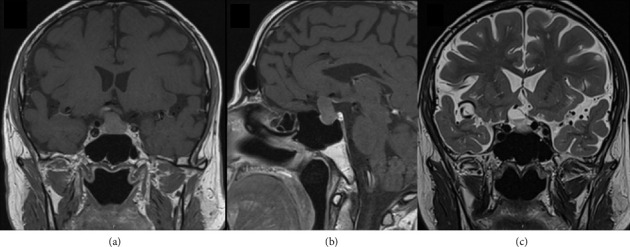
Preoperative MRI brain scan 10/2022. (a) Coronal T1-weighted image showing a hypointense pituitary mass compromising the left sinus cavernous. (b) Sagittal T1 showing the same pituitary mass growing through the diaphragm sellae. (c) Coronal T2-weighted image shows a hyperintense cystic pituitary mass.
